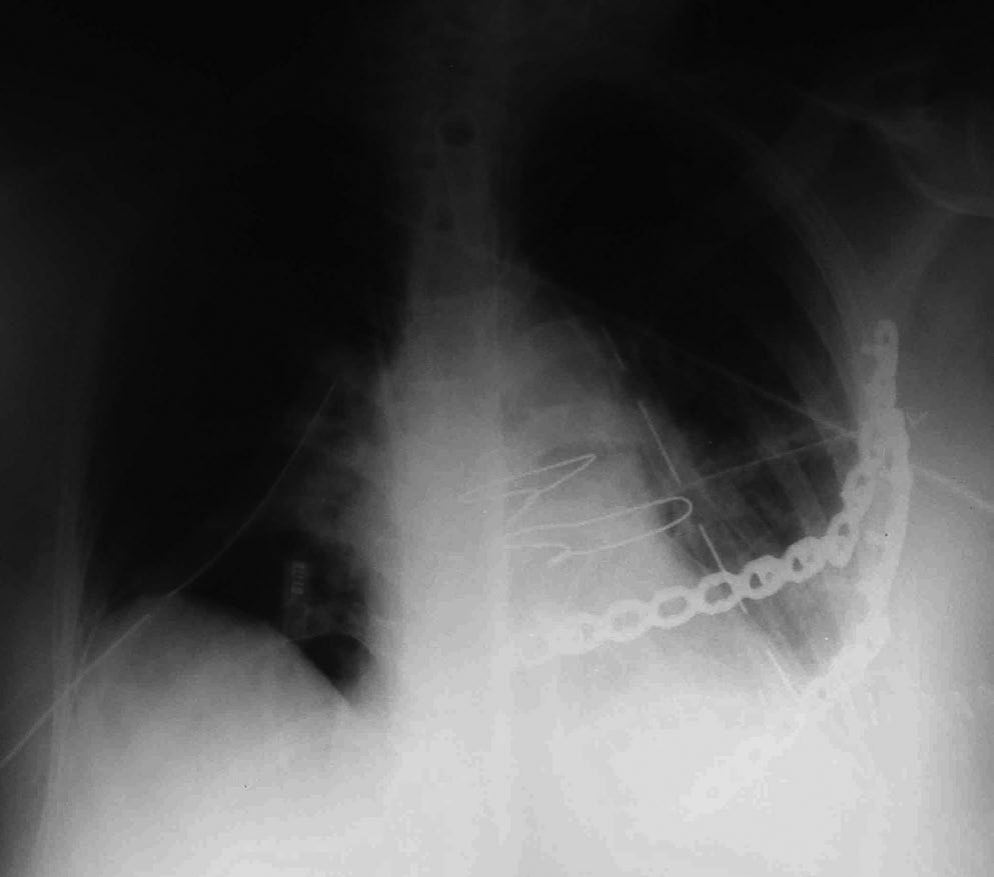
Postoperative radiograph showing the second and third costal cartilages approximated with sternal wires, and the fourth and fifth ribs fixed with reconstruction plates and screws
